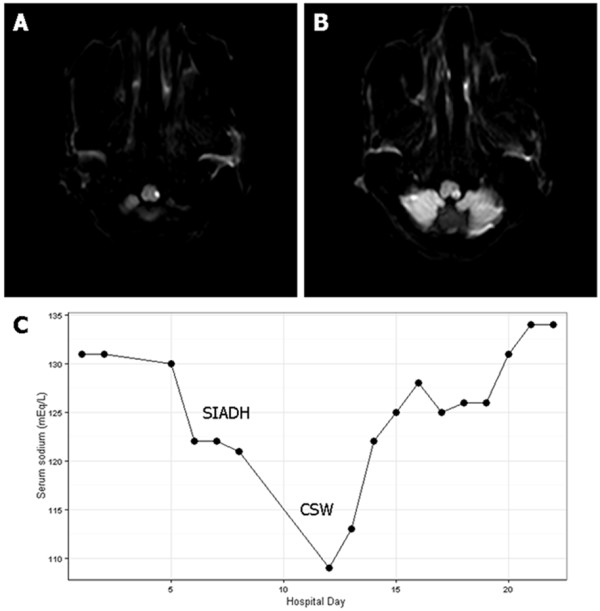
Brain magnetic resonance imaging and serum sodium level. Diffusion weighted image on admission showed left lateral medullary infarction (A, B) and serum sodium level after admission (C) demonstrated hyponatremia due to syndrome of inappropriate secretion of antidiuretic hormone (SIADH), and fluid restriction aggravated hyponatremia, suggesting cerebral salt wasting syndrome (CSW) as a final diagnosis.
